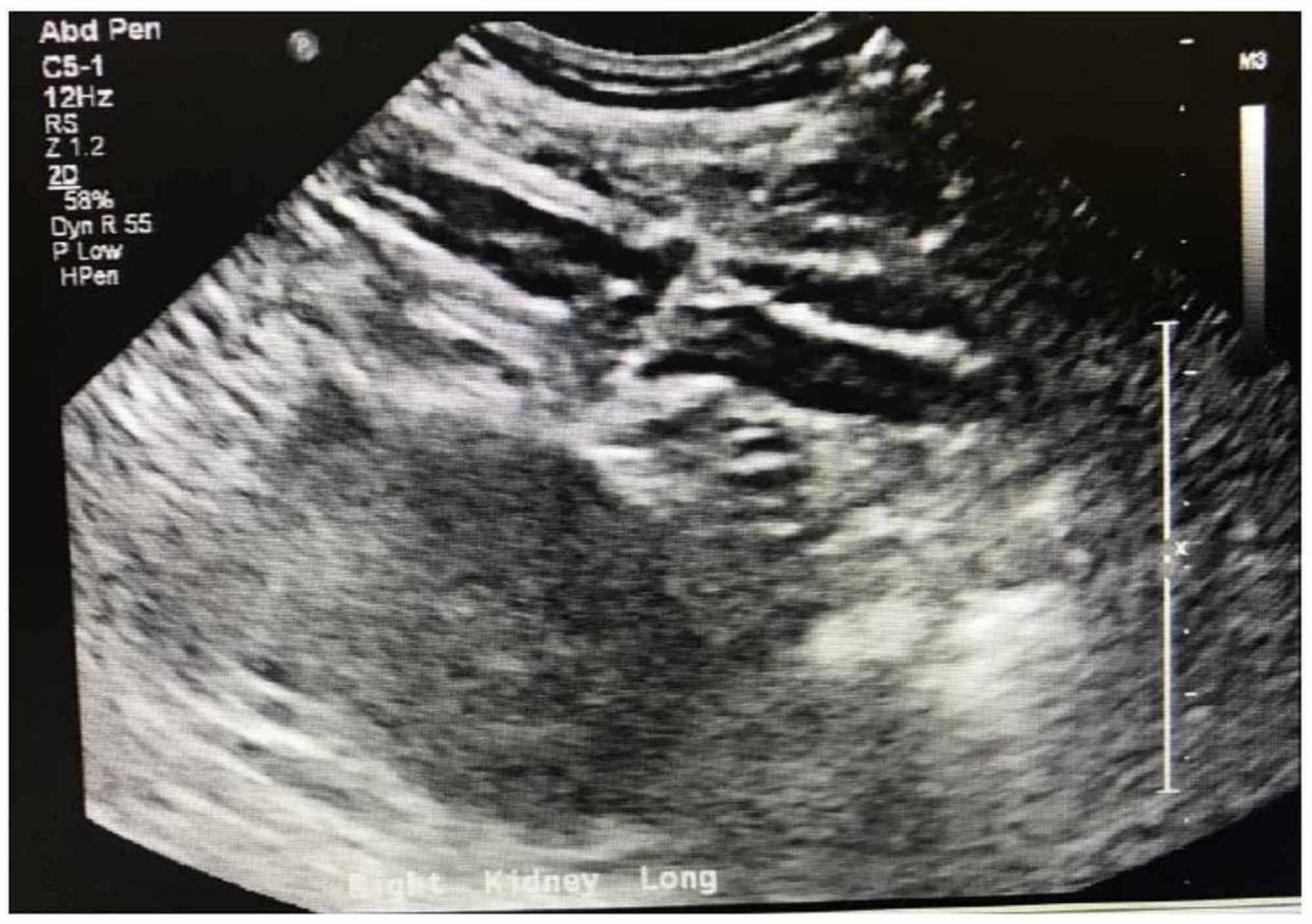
Ultrasound-guided needle biopsy of the right kidney
